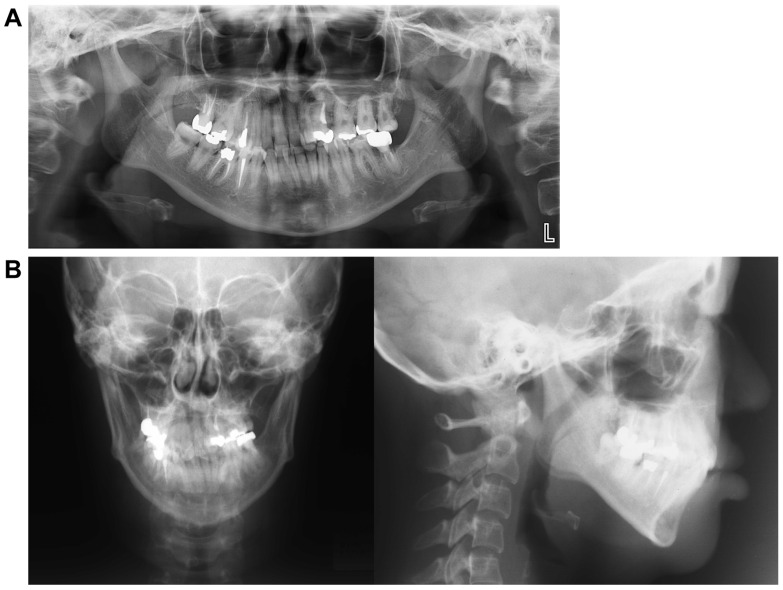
Pre-treatment record. (A) Panoramic radiograph. (B) Lateral and front cephalogram.
